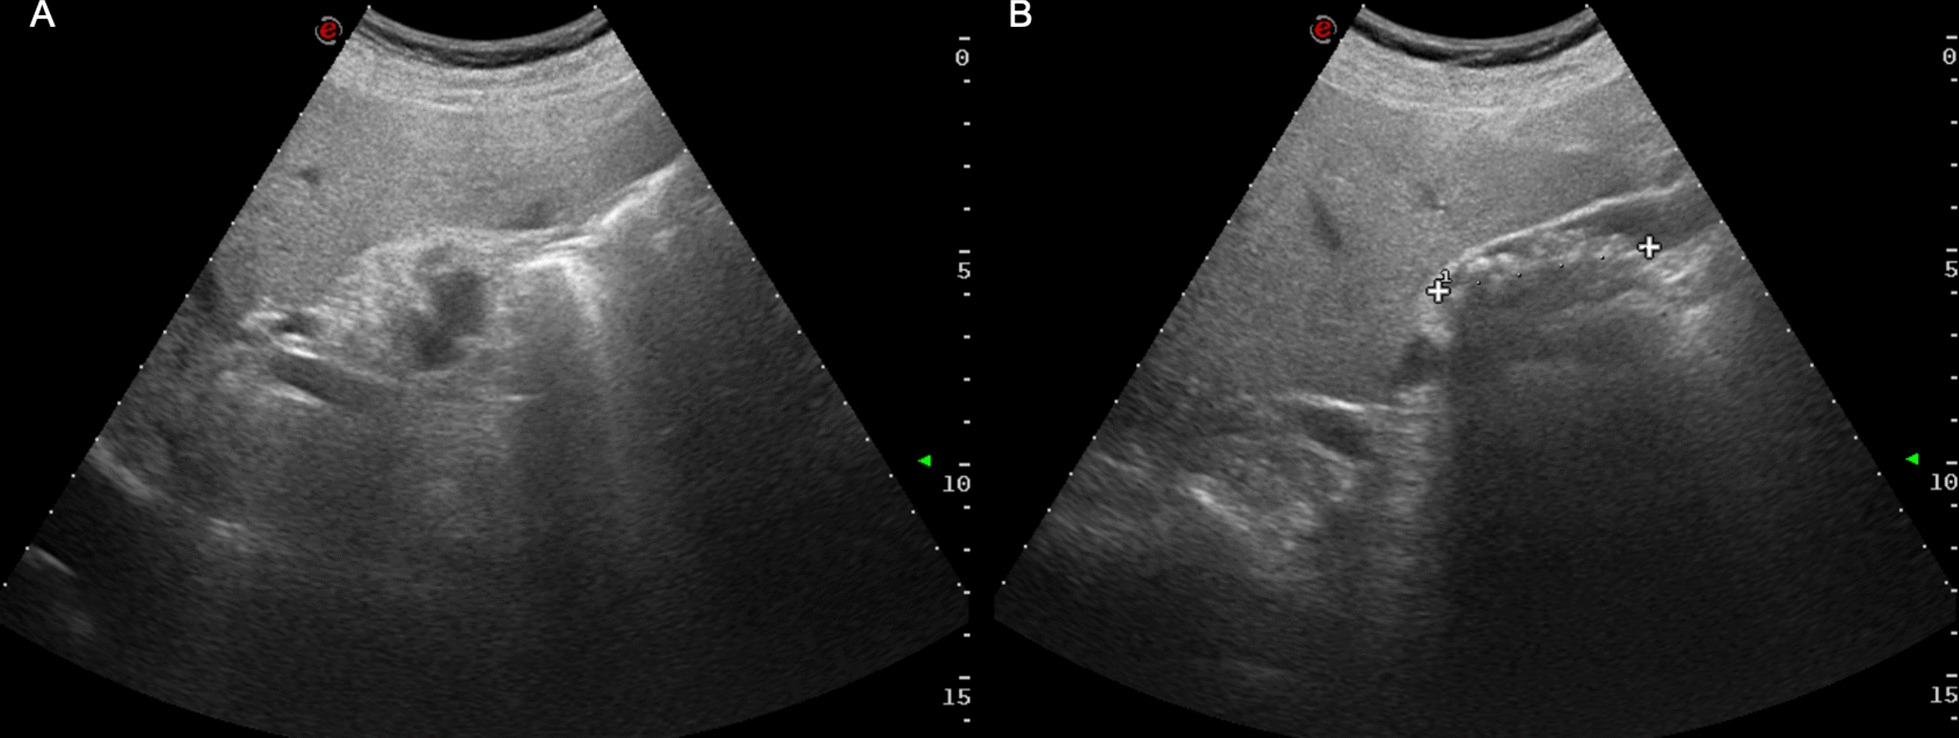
Pre-operative abdominal ultrasound. The dimensions of the liver are at the upper limit of normal, and it presents with rounded margins and diffusely increased echogenicity, findings diagnostic for hepatic steatosis. In this background, in the seventh liver segment, there is a hypoechoic area, measuring 12 mm in diameter, suggestive of hepatic hemangioma (Panel A). The intra-hepatic and extra-hepatic biliary ducts are normal. The gallbladder contains a very small amount of liquid, and its lumen is filled by a lithiasic agglomerate, measuring 5 mm in its maximal diameter (Panel B). The gallbladder walls are mildly thickened, with a concomitant mild edema of the perivisceral adipose tissue
